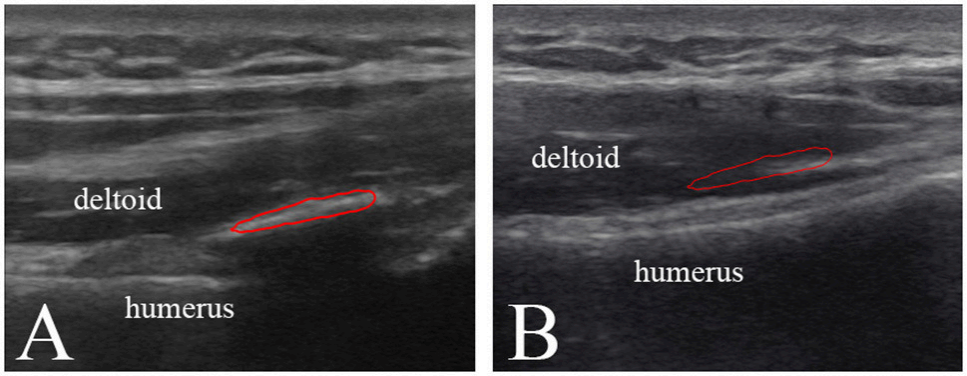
Ultrasound greyscale images of the injured left shoulder joint (A) and the uninjured right shoulder joint (B) of participant No. 2. Red profile in (A) and (B) represents the HO segmented from the surrounding soft tissues and the normal muscle tissue selected on the corresponding position, respectively.
